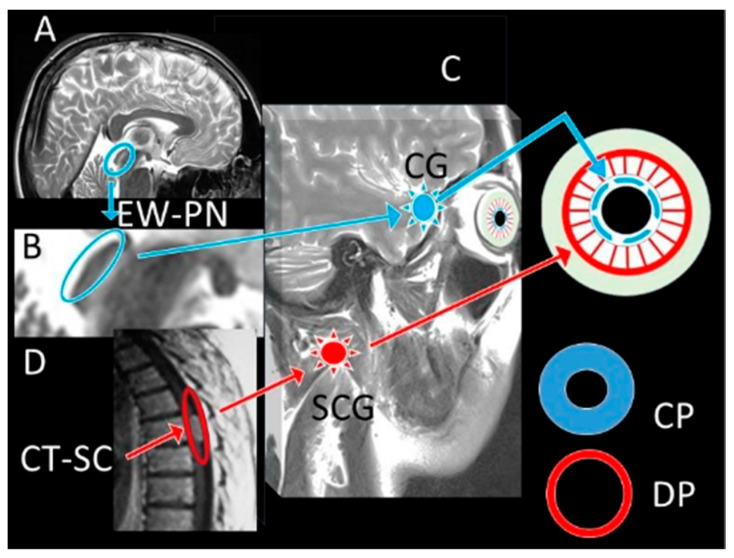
Table of Pathogenesis: Pathogenesis Table: Human brain MRI; the location of the Edinger Westphal and Perlia nuclei (EW-P) located in the tegmentum mesencephali in the superior colliculus (A,B), their connection with the ciliary ganglion network (CG) and pupillary muscles (Blue arrows), the location of the parasympathetic pupilloconstrictor fibres and muscles affecting the pupilloconstrictor muscles that innervate the pupillae (Blue/(A,B)) (C); the postganglionic fibres going to the pupillodilator muscles (Red arrow/(B)) of the sympathetic cervical ganglion network (SCG) originating from the cervicothoracic sympathetic centre (CT-SC) and coming to the superior cervical sympathetic ganglion (SCG), and the pupillodilator muscles they affect are observed (D). While the pupils contract with the parasympathetic effect (CP), they dilate with the sympathetic effect (DP). Representative constricted pupil (Blue Ring) and dilated pupil (Red Ring) are seen.
